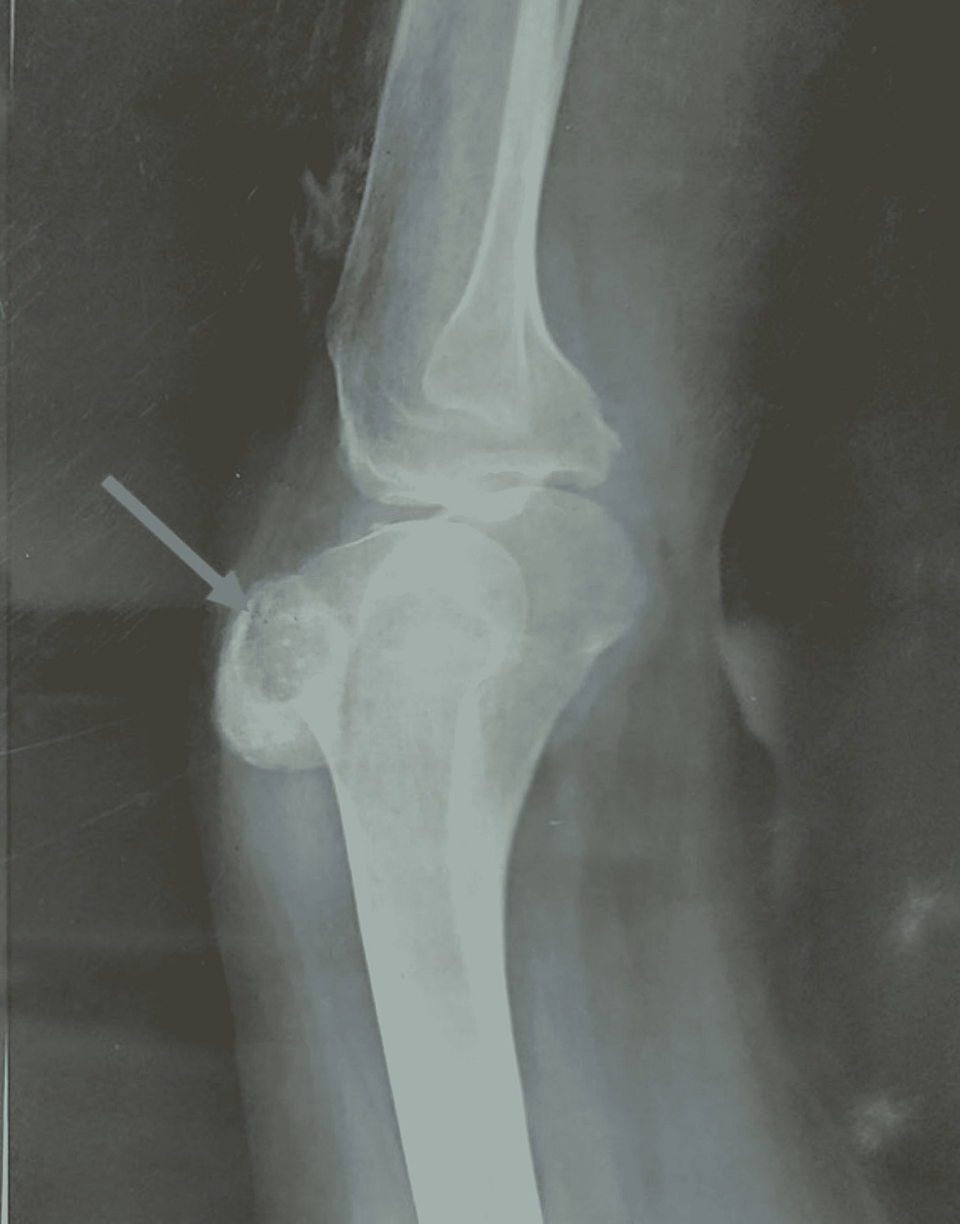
Lateral X-ray of the knee demonstrating localized lytic lesion in the patella with soft-tissue swelling overlying.
